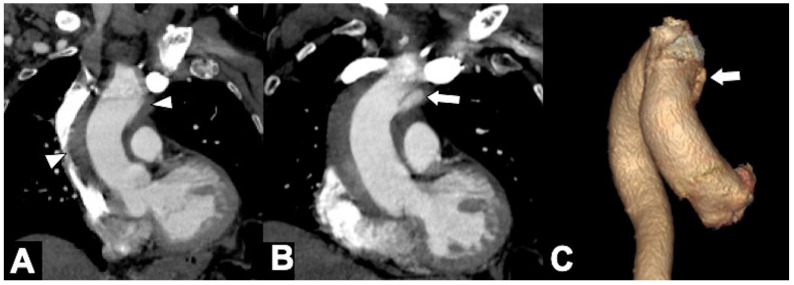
Unstable Type A IMH complicated by ulcer-like projection (ULP). (A) Coronal oblique CTA shows type A IMH (arrowheads) in a 68-year-old man with hypertension and chest pain. The total aortic diameter was less than 50 mm and the IMH thickness less than 11 mm; he underwent medical management initially. (B) Three-day follow-up coronal oblique CTA image shows disease progression by an ULP due to opening of intimal tear (arrow). (C) 3D-volume-rendered (VR) reconstruction confirms ULP (arrow).
